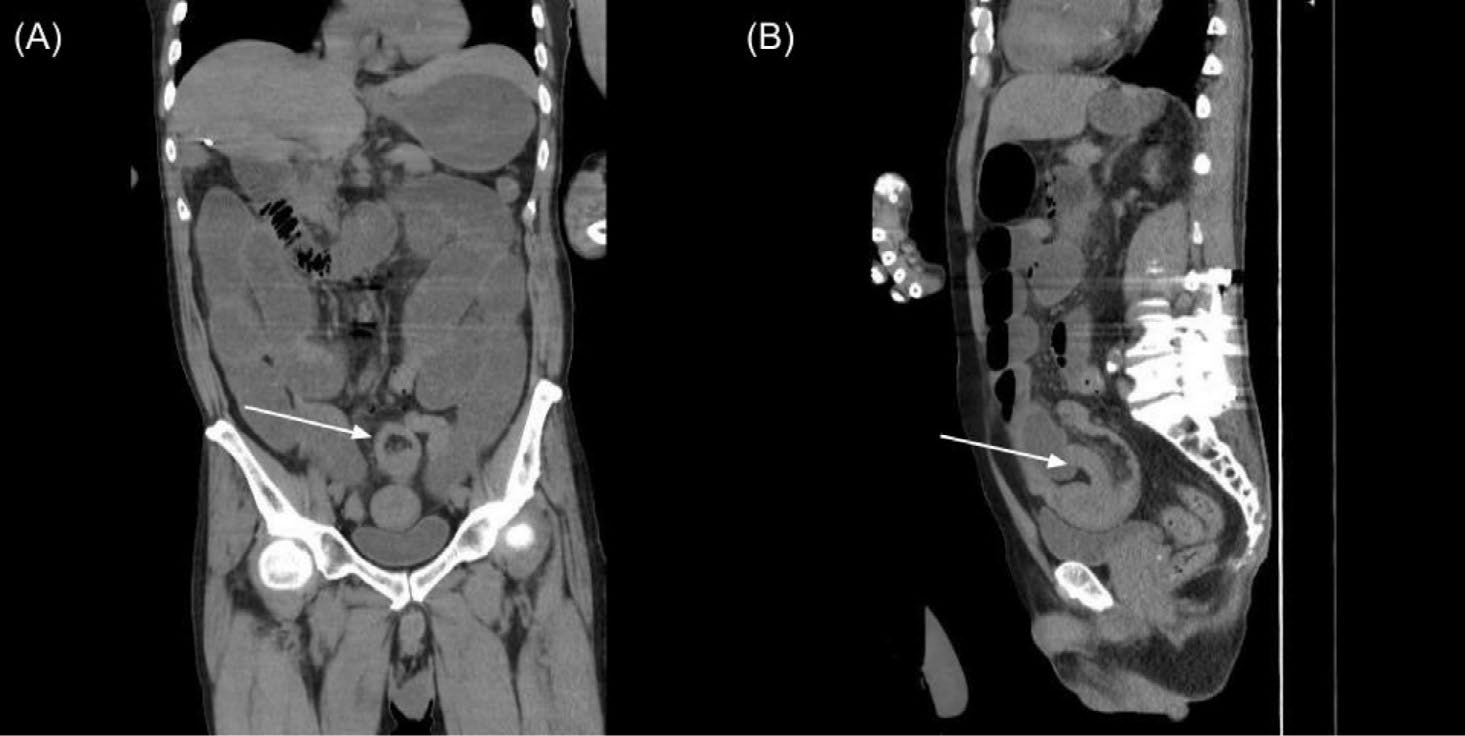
The preoperative computed tomography images show diffuse small bowel ileus with distal ileum intussusception (white arrow) in (A) the coronal view and (B) the sagittal view.
